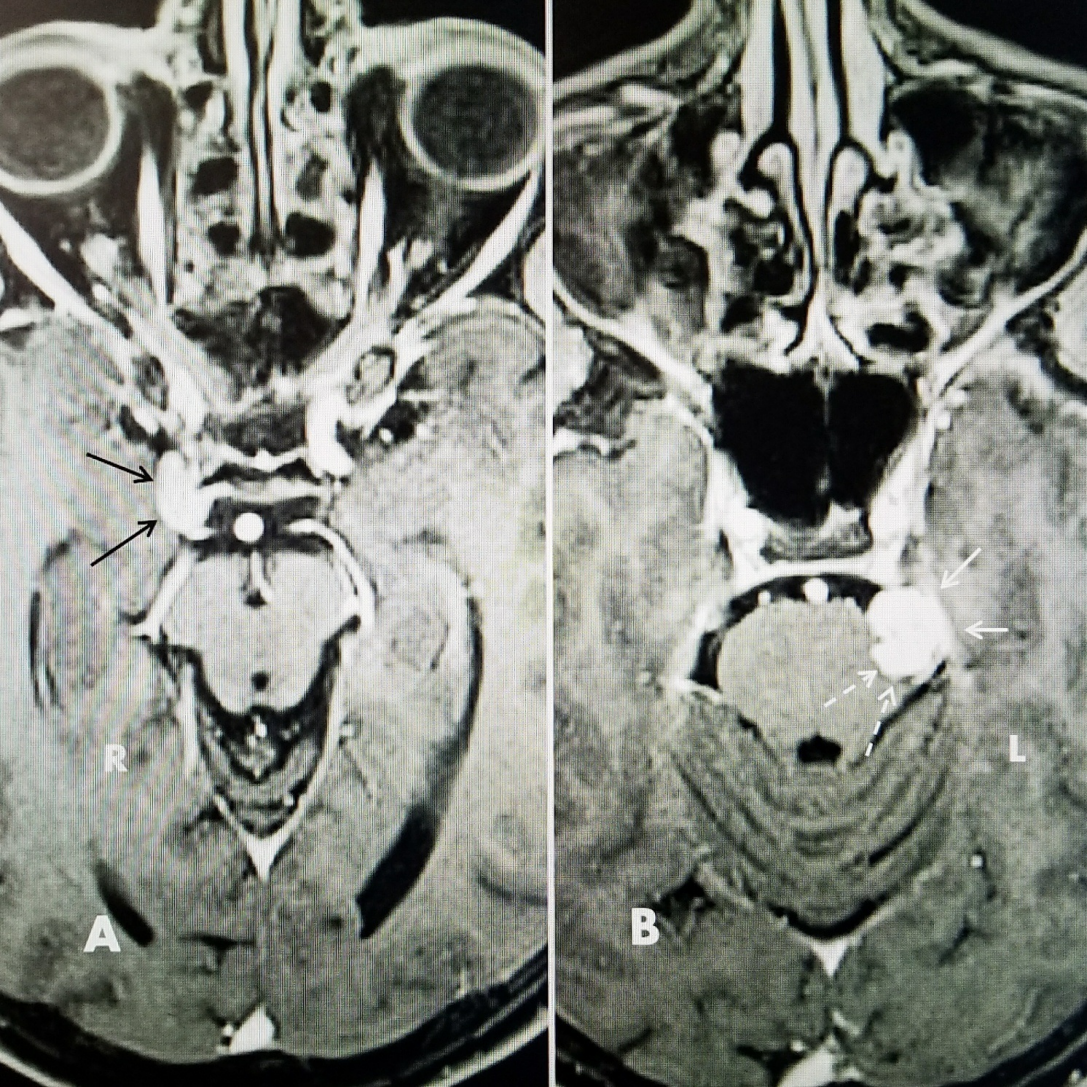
Magnetic resonance imaging T1 MRI with gadolinium showing right cavernous sinus meningioma and left tentorial notch tumor. A: Axial T1 MRI with contrast: showing right (R) cavernous meningioma (black arrows). B: Axial T1 MRI with contrast showing left (L) bilobed irregular tentorial mass indenting pons in left prepontine and trigeminal cistern. White arrows show smooth attachment to tentorium. The dotted white arrows medially show the relationship of the tumor to trigeminal root entry zone when the patient had no trigeminal pain.
